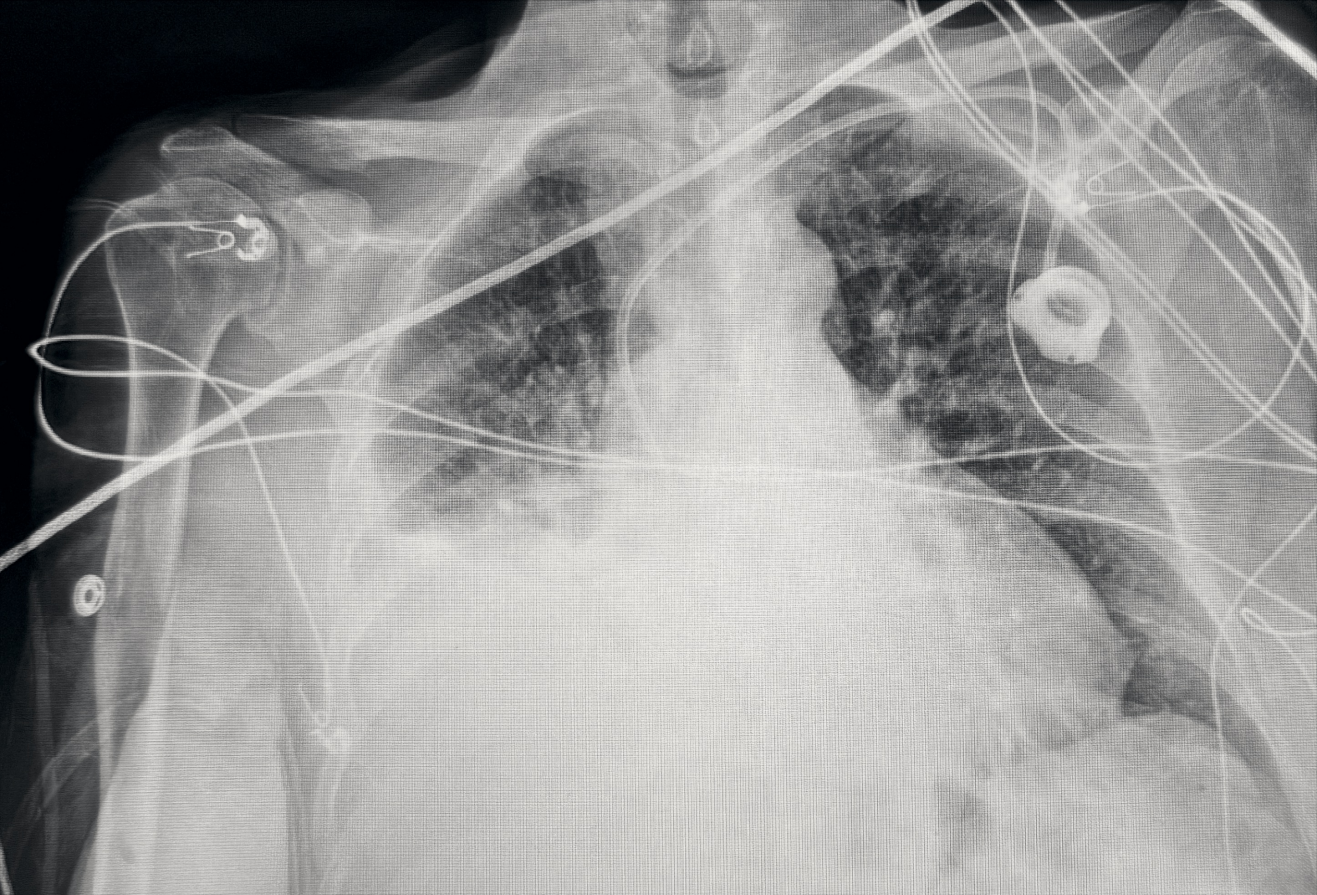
Final post-operative chest X-ray after replacement.
